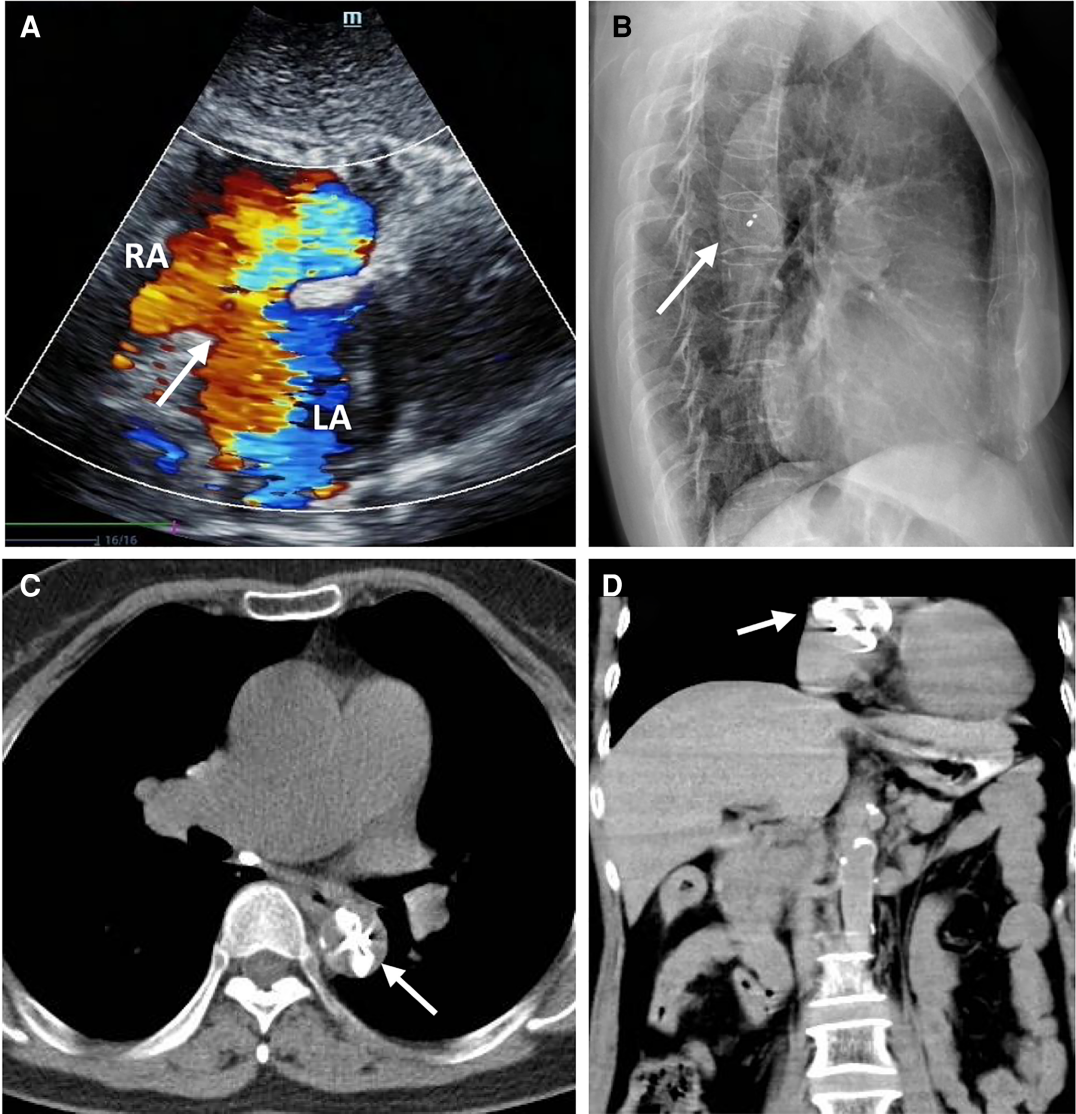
The ASO device embolization. Transthoracic echocardiography showing no occluder device and an interrupted echo (white arrow) in the middle of the atrial septum associated with a large number of left to right shunt flow (A); The lateral chest x-ray showed that the embolized occluder was located in the descending aorta (white arrow) (B); The chest CT showed that the embolized occluder device (white arrow) was located at the descending thoracic aortic isthmus (transverse view, C), (coronal view, D).
